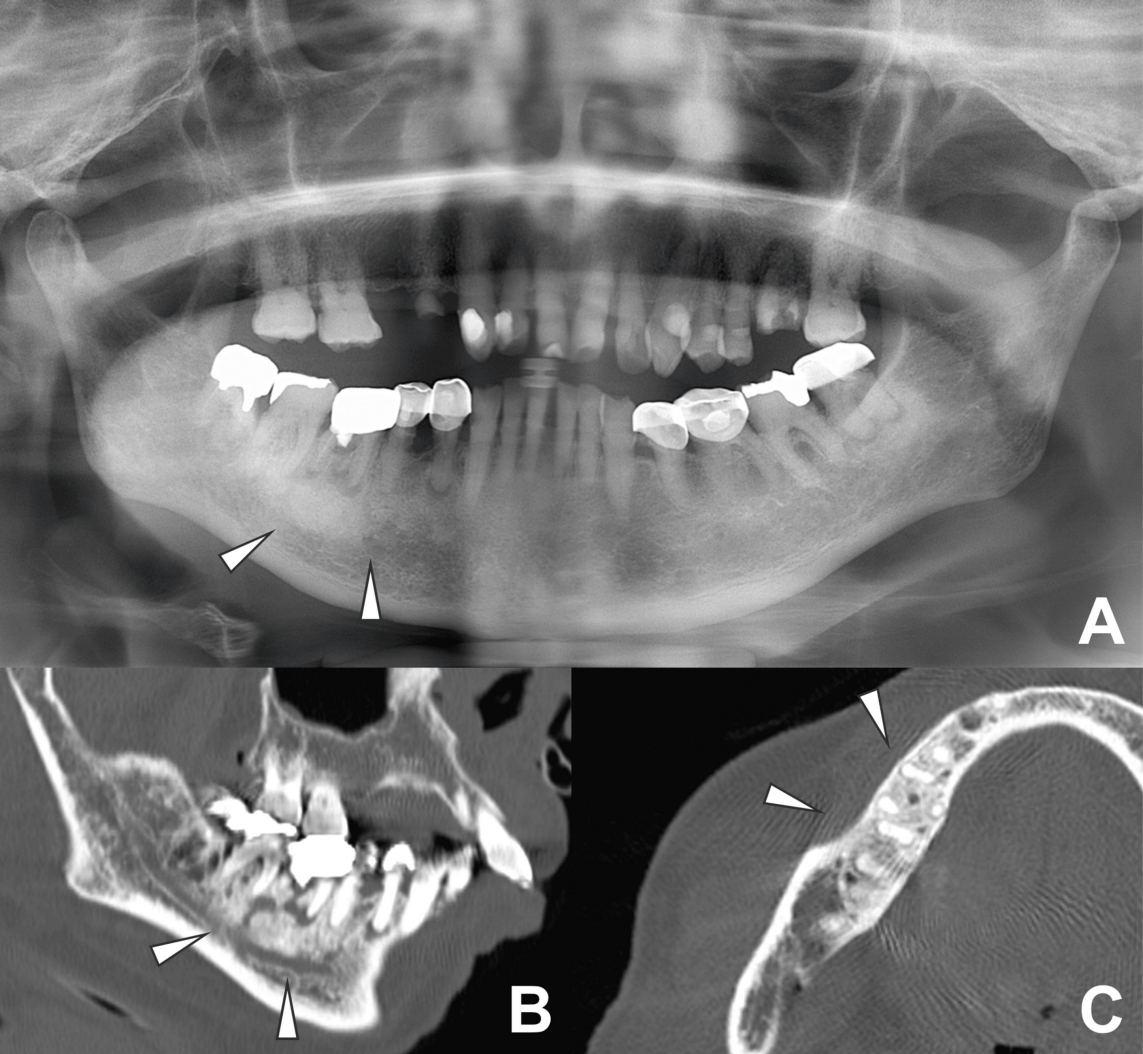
Panoramic radiograph and head CT images A: panoramic radiograph indicates sclerosis of the right mandibular bone (arrowheads); B, C: head CT images indicate a periapical lesion at the root of the right molar, with significant surrounding sclerosis (arrowheads).
